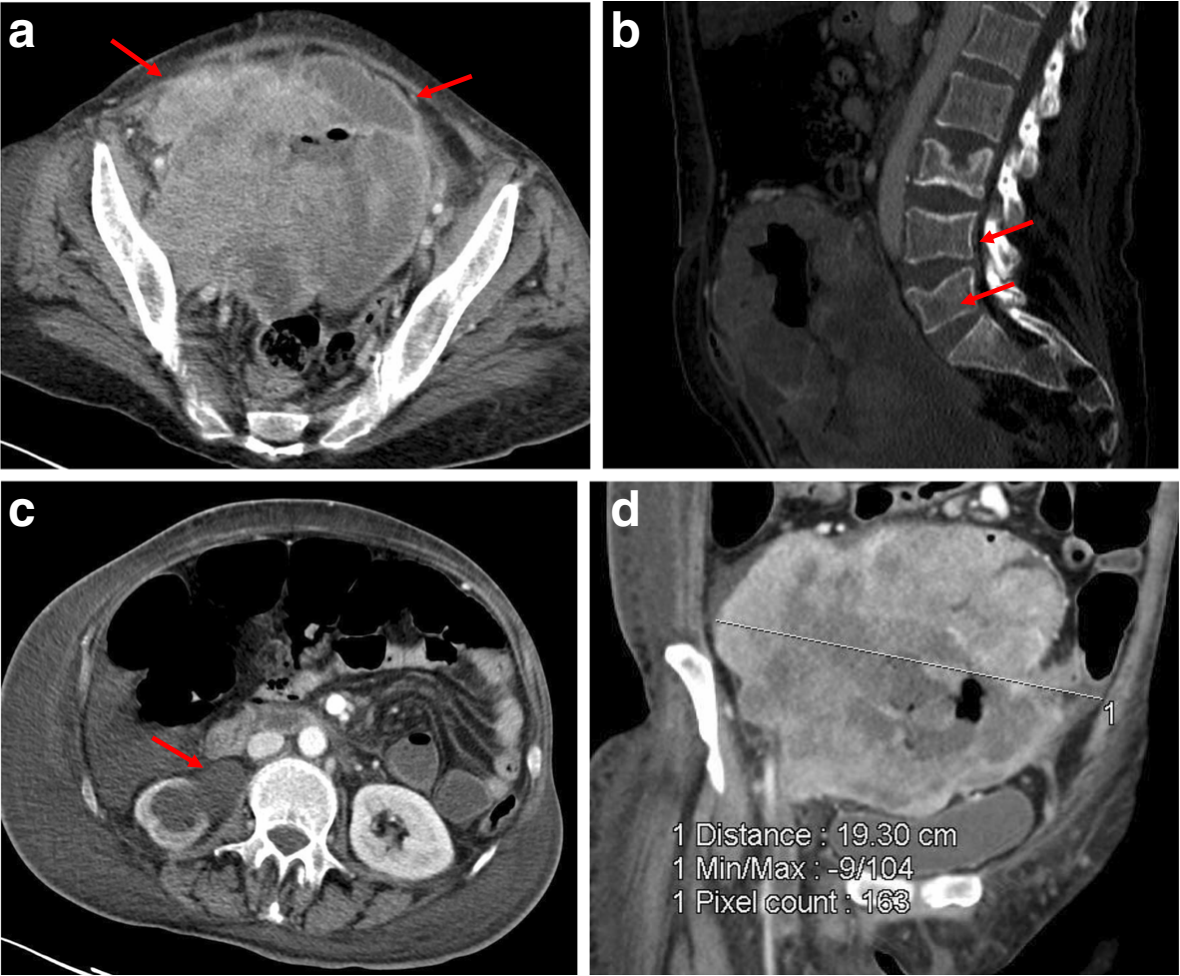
a Computed tomography scan at diagnosis showing the pelvic mass (*arrows*). b Computed tomography scan at diagnosis showing the bone metastasis in L3 (*arrows*). c Computed tomography scan at diagnosis displaying a right hydronephrosis (*arrows*). d Computed tomography scan at diagnosis showing the pelvic mass
